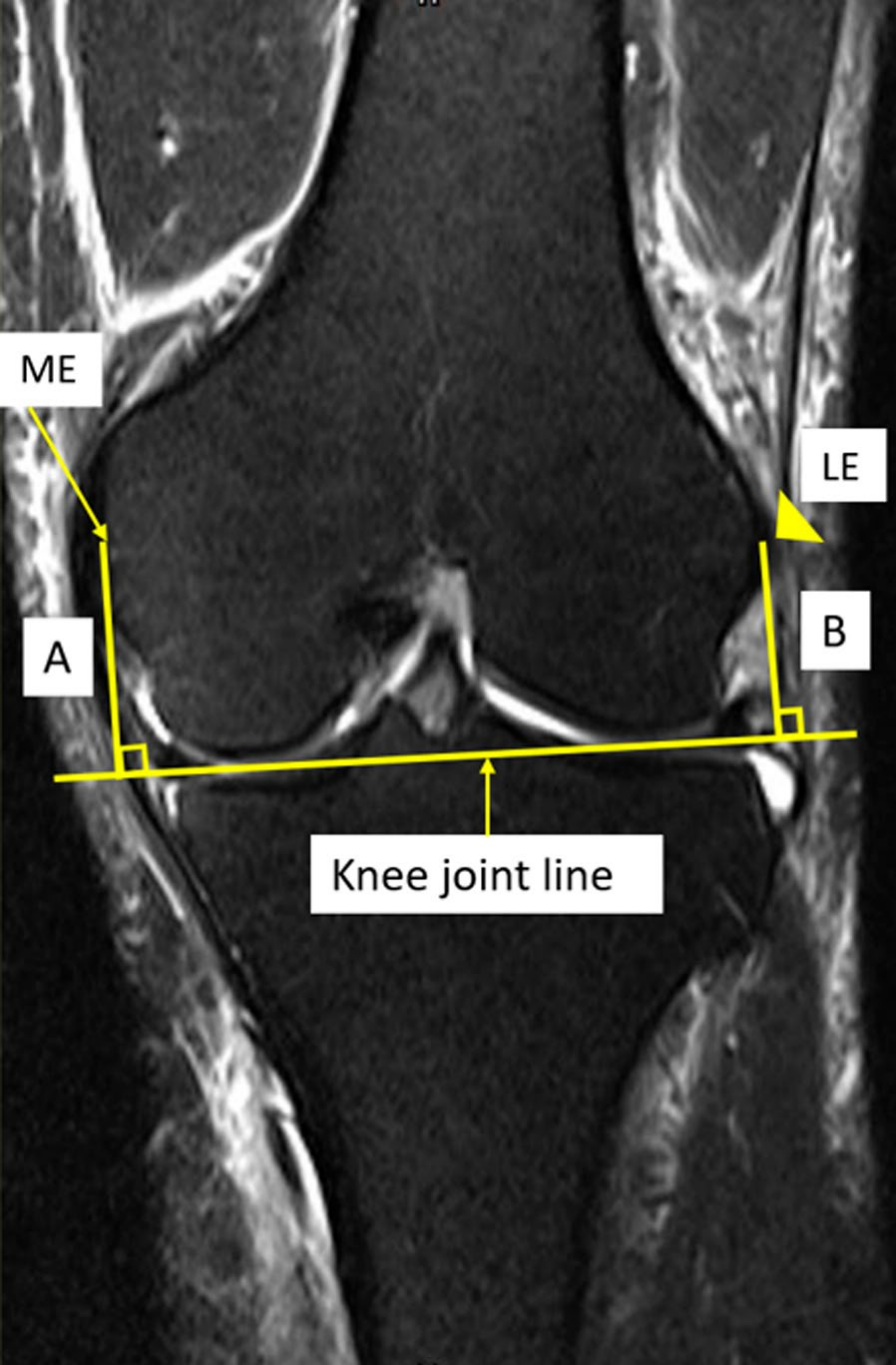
Demonstration of the measurement technique in coronal view of MRI. The knee joint line connects the most distal point of medial femoral condyle and lateral femoral condyle. Line *A* is the distance from the most prominent point of medial femoral epicondyle (ME, arrow) perpendicular to the knee joint line. Line *B* is the distance from the most prominent point of lateral femoral epicondyle (LE, arrowhead) perpendicular to the knee joint line
